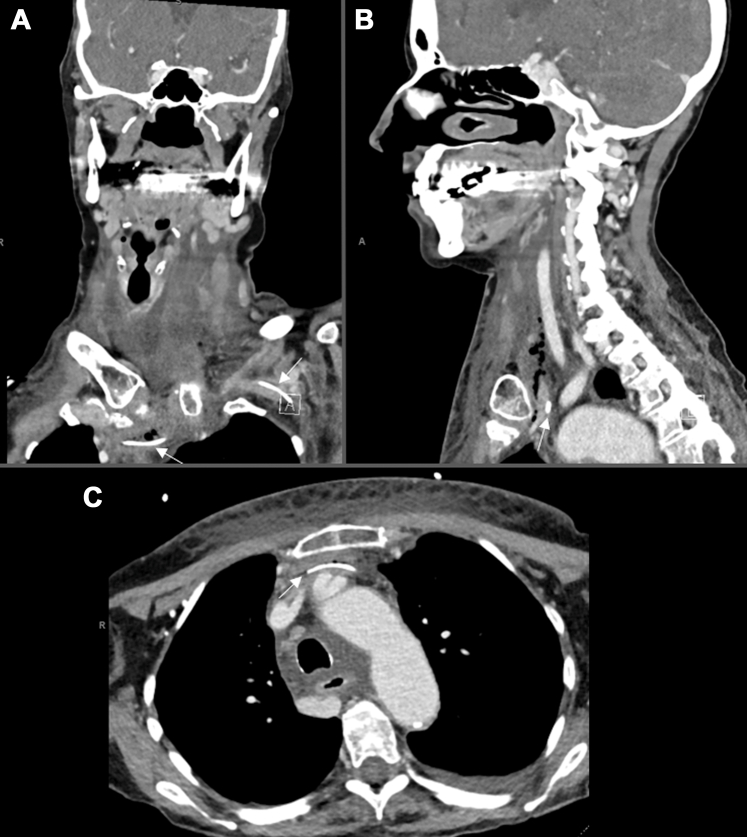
Computed tomography scan shows left upper extremity peripherally inserted central catheter (PICC) with tip terminating anterior to the left brachiocephalic vein (bottom arrow in A and arrow in C). Arrows are pointing to the PICC line. The top arrow in A shows the PICC line within the brachiocephalic vein. The tip of the PICC line is approximately 2 cm anterior to the confluence in the anterior mediastinum, with pneumomediastinum ascending along the left internal jugular vein (arrow in B) and low-density fluid in stranding throughout the anterior mediastinum, at the anterior chest subcutaneous soft tissues, and the deep spaces of the neck tracking superiorly.
